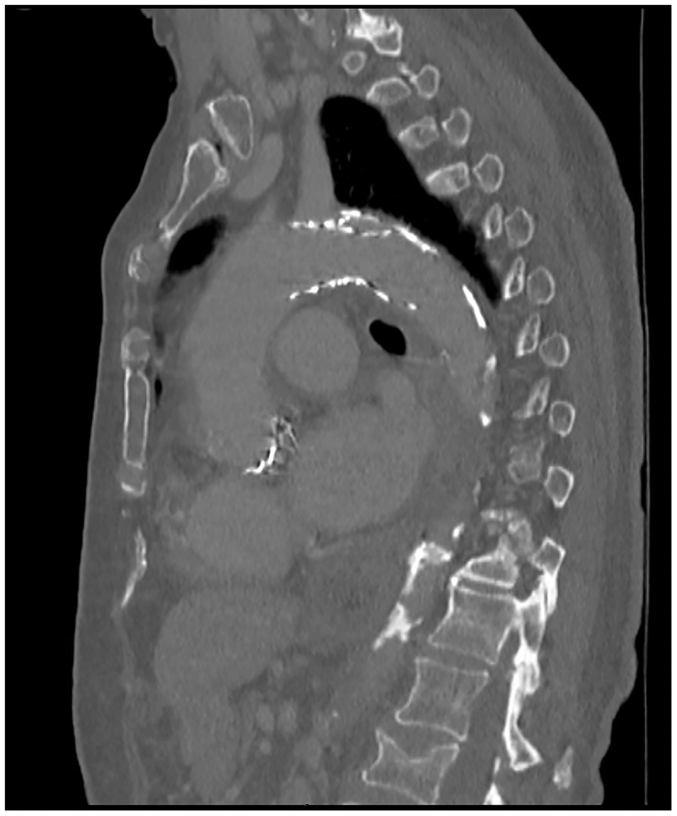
Sagittal view of computed tomography angiography (CTA) of the chest demonstrating intact transcatheter aortic valve replacement (TAVR) and patent aortic stent.
